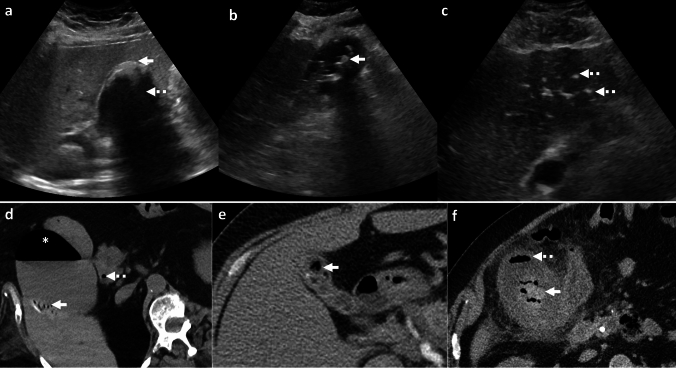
Pitfalls for emphysematous cholecystitis. a Wall echo shadow (WES) complex: Transverse US image shows chronic calculous cholecystitis and WES complex with thickened gallbladder wall (solid white arrow, a) and calculus with posterior acoustic shadowing (dotted white arrow, a). b, c Pneumobilia: Transverse US images b, c show echogenic air foci with dirty posterior acoustic shadowing within the lumen of the gallbladder (solid white arrow, b) and within the intrahepatic bile ducts (dotted white arrows, c). d–f Air-containing gallstones and pneumobilia in three different patients. CT image in (d) patient with trauma and abdominal pain shows distended gallbladder with gas in gallstones (solid white arrow, d), air-fluid level in gallbladder lumen (asterisk, d), and air in common bile duct post sphincterotomy (dotted white arrow, d). CT image of another patient e shows a collapsed gallbladder with incidental gas in gallstones (solid white arrow, e). CT after ERCP stenting in a patient with known acute cholecystitis f shows an air-fluid level in the gallbladder lumen (dotted white arrow, f) and air mixed with sludge and calculi in the gallbladder lumen (solid white arrow, f). There is no air in the gallbladder wall to suggest emphysematous cholecystitis
